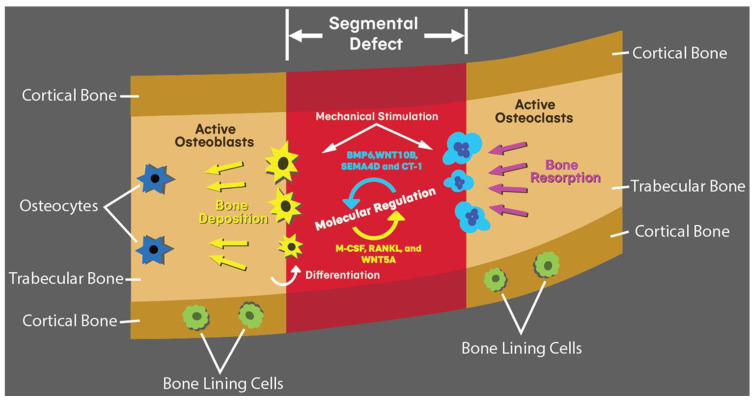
Actions of osteoclasts, osteoblasts and bone lining cells in the context of a segmental mandibular defect. Selected molecular mechanisms of regulation between osteoblasts and osteoclasts include BMP6, WNT10B, SEMA4D, CT-1, M-CSF, RANKL, and WNT5A.
